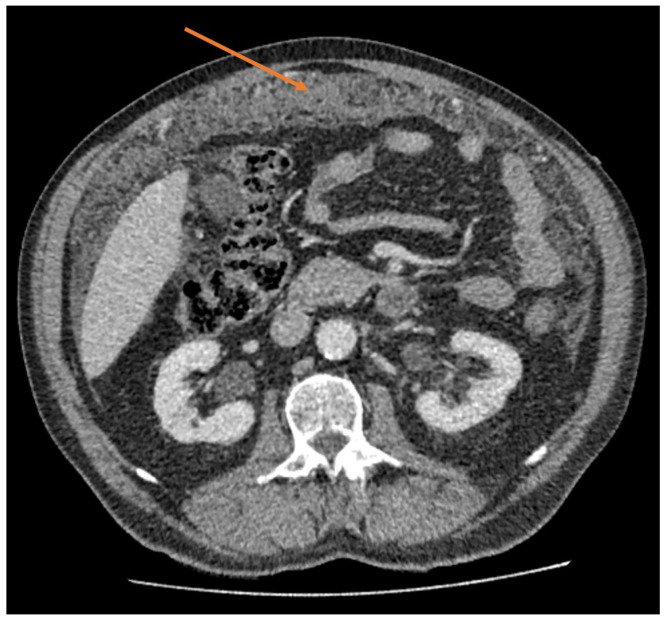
Abdominal CT scan (axial view) demonstrating significant diffuse thickening of the greater omentum (orange arrow), also known as “omental caking”.
